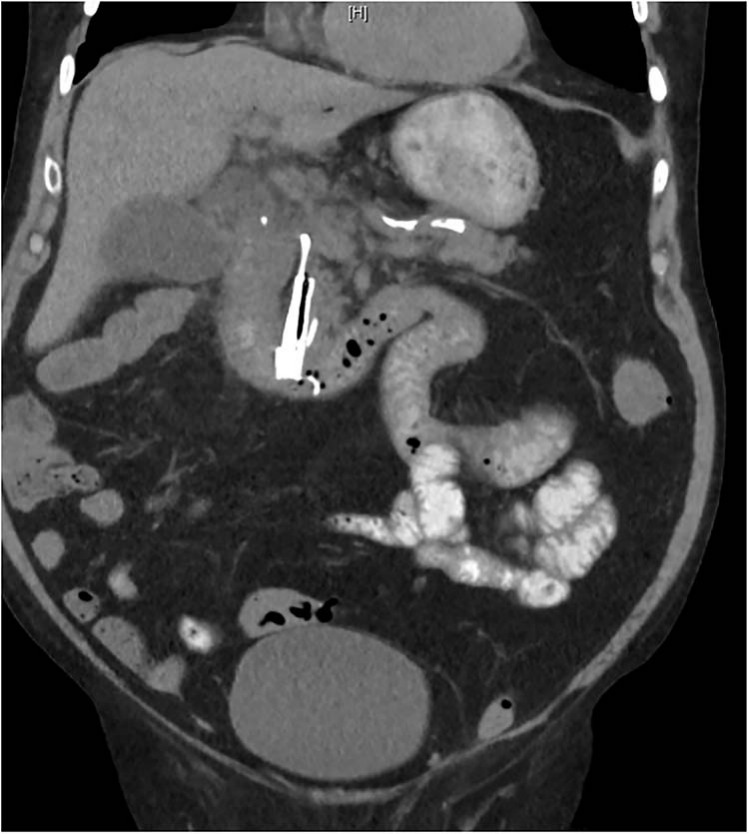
Abdominal CT showing a metallic biliary stent in distal CBD.
